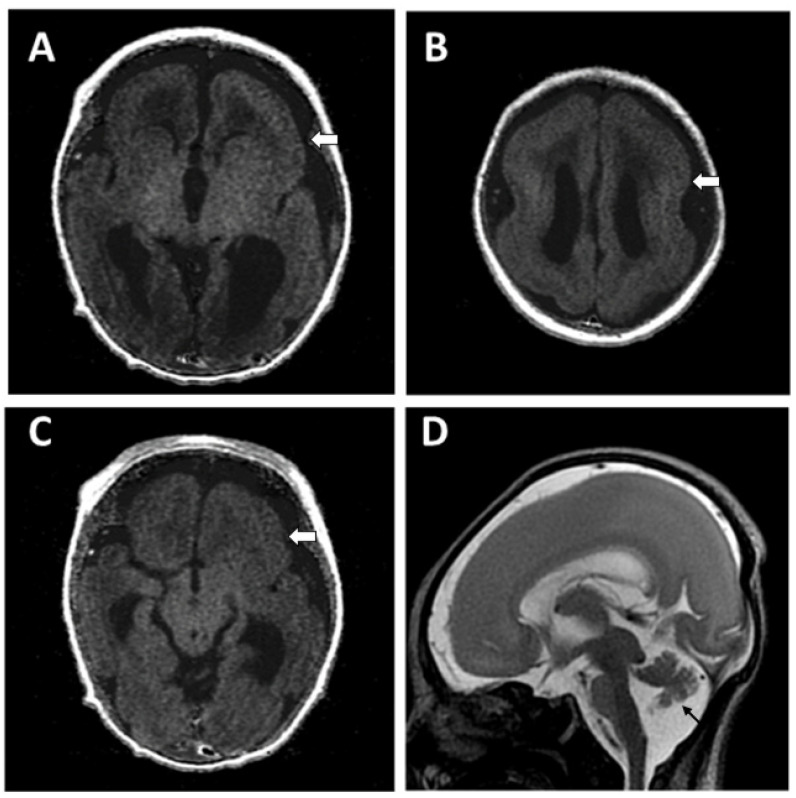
Brain MRI of Case 2 showed lissencephaly (white arrows), mildly dilated posterior horns of ventricles with dysgenesis of carpus callosum, and small vermis (arrow) ((A–C): T1WI, (D): T2WI).
